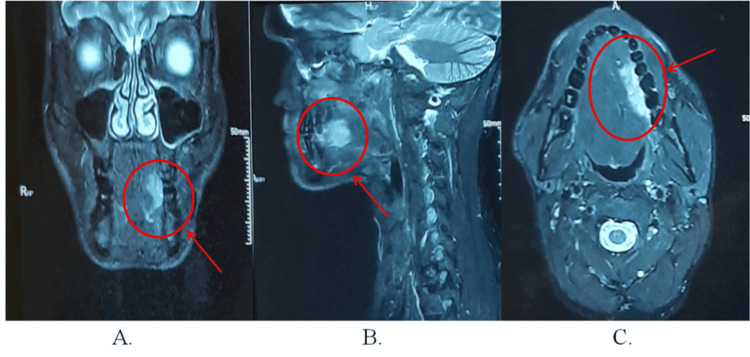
Preoperative radiographs of patient 1 (A) Coronal section of CE-MRI scan (face), (B) sagittal section of CE-MRI scan (face), and (C) axial section of CE-MRI scan (face).
